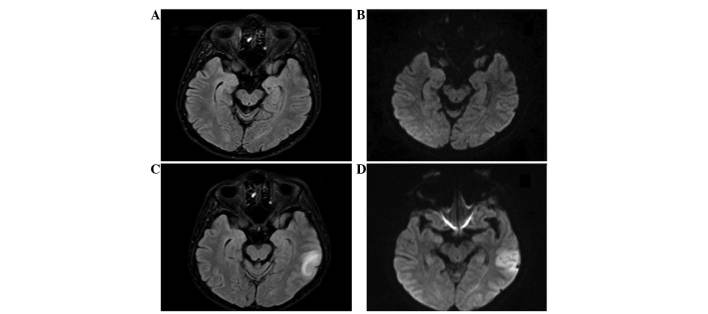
(A and B) Normal cranial magnetic resonance imaging and (C and D) flair and diffusion weighted images showing acute left parietal infarction.
